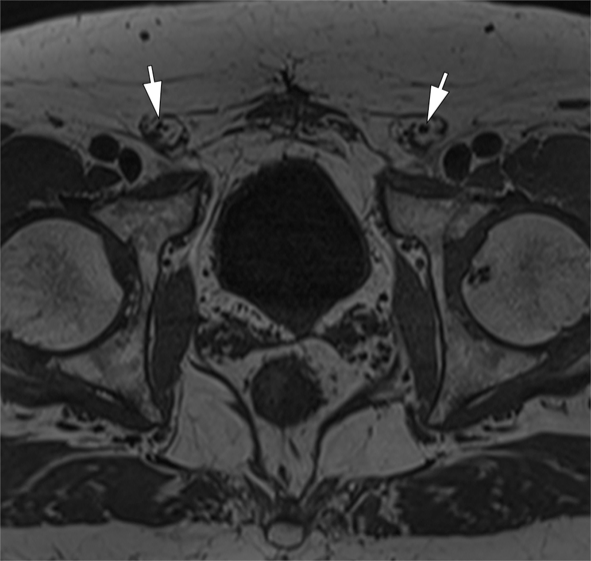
Genitofemoral nerve. Axial 3D volumetric T1-weighted image at the level of the hips demonstrates the normal genitofemoral nerves within the inguinal canals (arrows)
